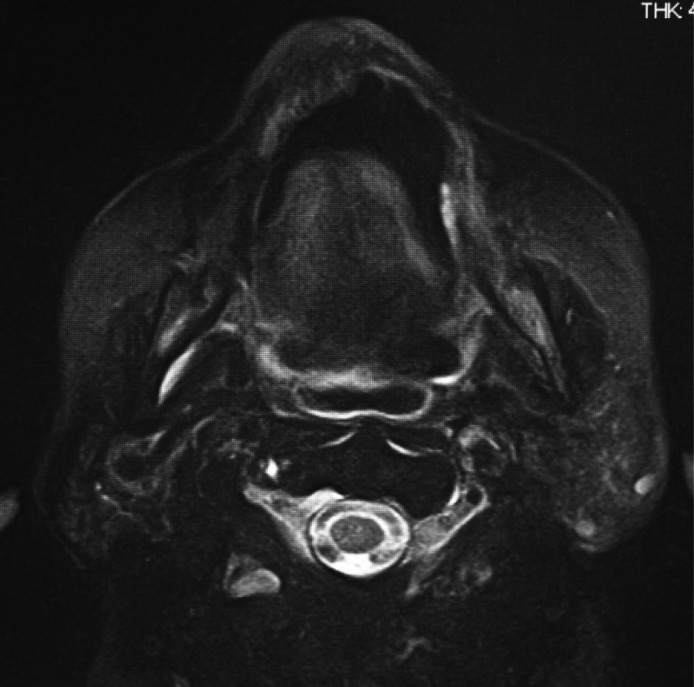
A T2‐weighted magnetic resonance image showing a lymphatic malformation with a clearly distinguishable outline of the buccinator muscles
